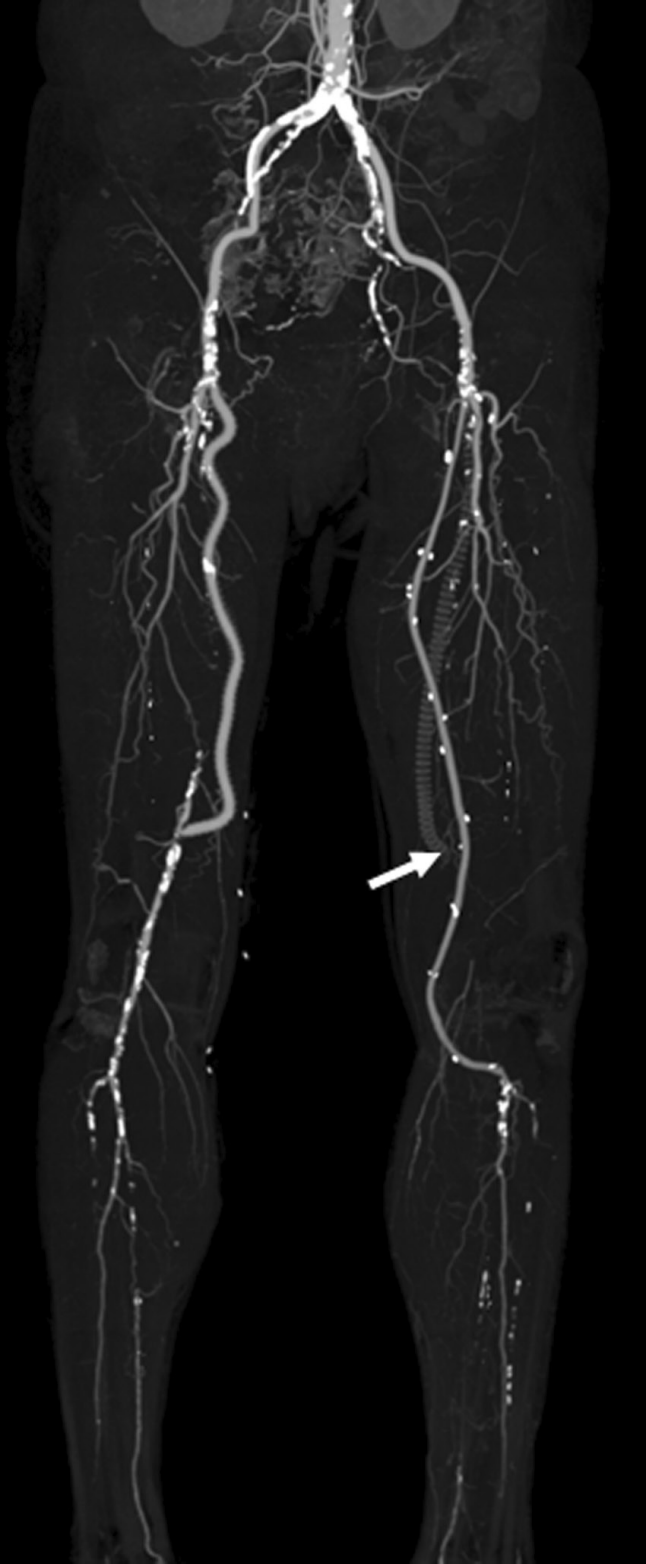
Postoperative computed tomography reveals patency of the bypass graft (arrow) with no evidence of embolism.
